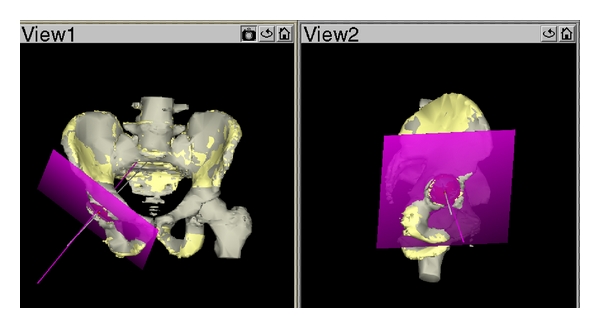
Example of a CT-scan validation process, where both models (pre- and postoperative) are superimposed to calculate the cup orientation based on the coordinate system defined preoperatively.
